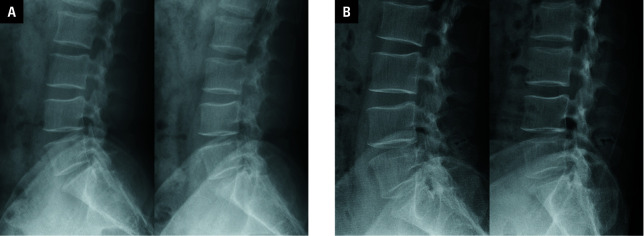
Dynamic X-ray images of a 38-year-old man with huge lumbar disc herniation before (A) and after surgery (B) showing improvement in the range of motion at the L4–L5 level from 3.5 to 3.7 °. The position of the IntraSPINE device was appropriate and stable in dynamic positions (arrow).
